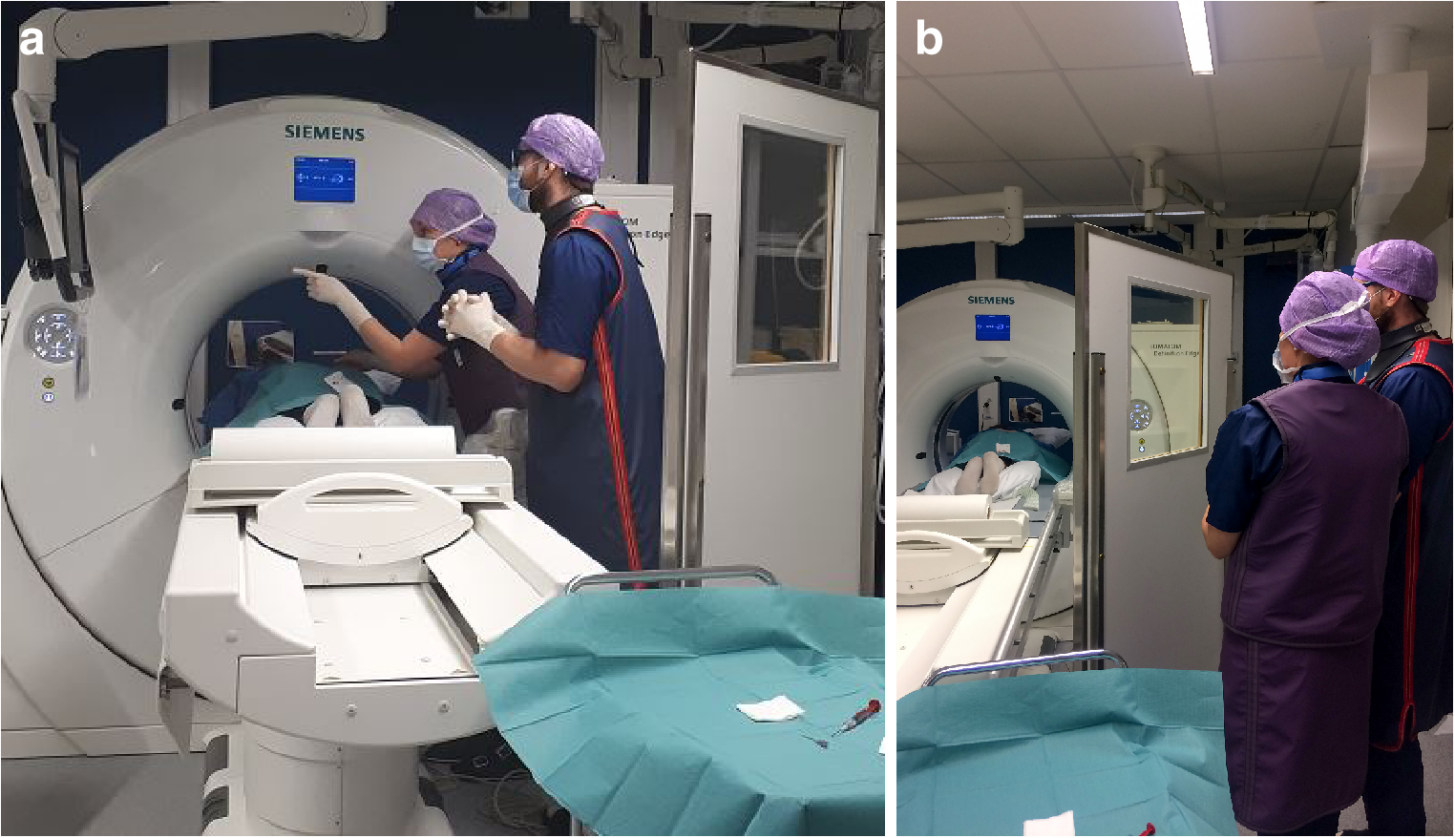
A typical positioning of the operators during a CT guided operation where (a) the operator needed to hold the instruments and stay approximately 30–50 cm from the fluoroscopy area and (b) the operators were able to stand behind the lead wall during fluoroscopy without having to constantly hold the instruments. The operator is on the left and the assisting operator on the right. In this particular procedure, the operator is not wearing lead glasses, the assistant operator is
